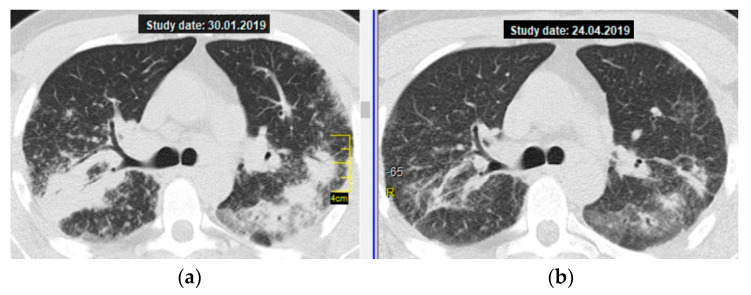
Pulmonary sarcoidosis, stage II, atypical form—multiple massive consolidations in both lungs; a thoracic CT scan (axial section at the level of the tracheal bifurcation): (a) on the left—before treatment, (b) on the right—regression after 3 months of MTX therapy (15 mg/week).
